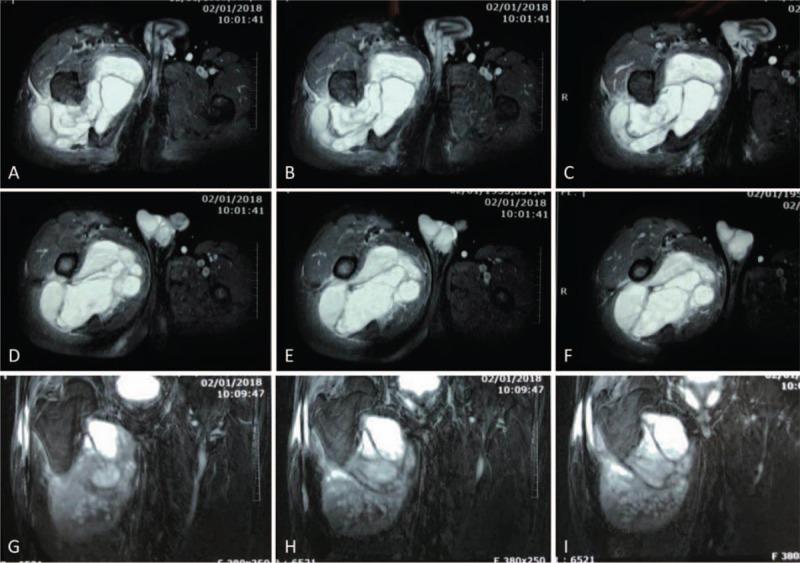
(A–I) Preoperative coronal and transverse MRI scan revealed the irregular mass in his right thigh.
